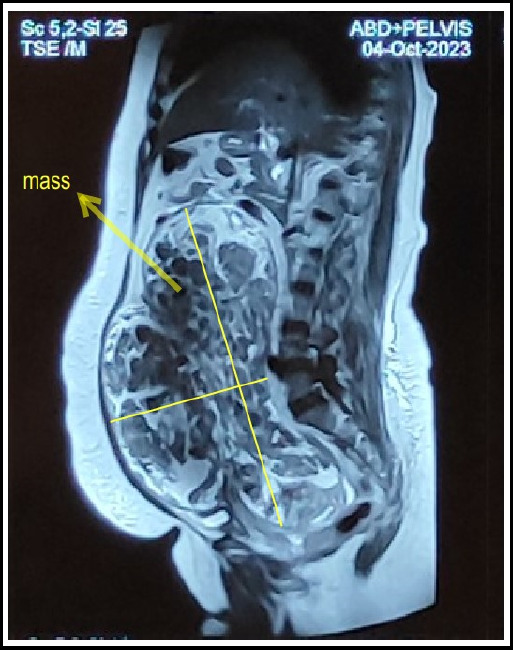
MRI Sagittal view of abdomen-pelvis showing thin walled, capsulated lobulated solid mass.
